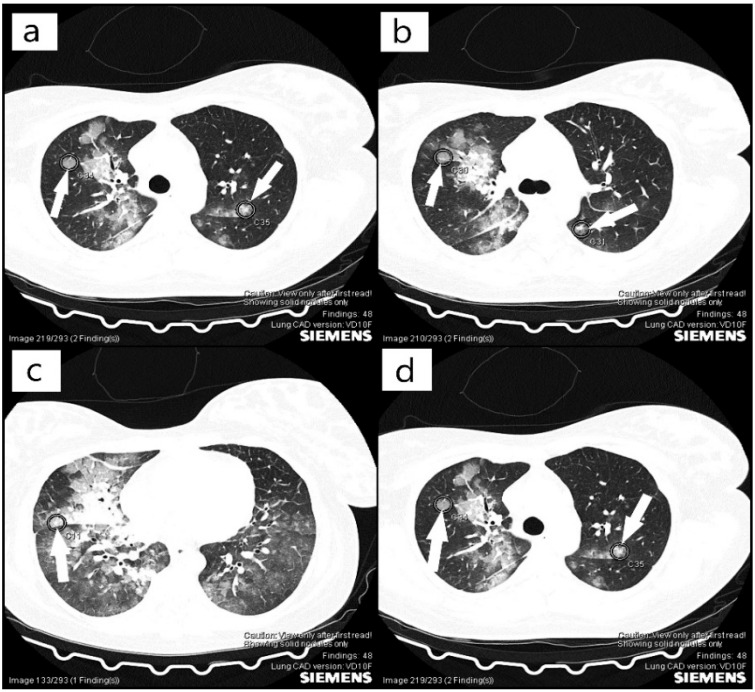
(a–d) Computer tomography (CT) scan with pulmonary microthrombosis (arrows).
